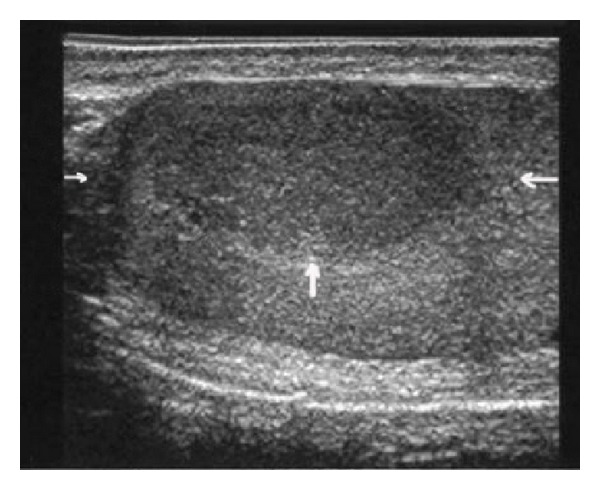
Scrotal sonogram showing a nodular lesion in right testicle (arrows). The lesion is homogeneously hypoechoic, well demarcated, and associated with distal enhancement.
